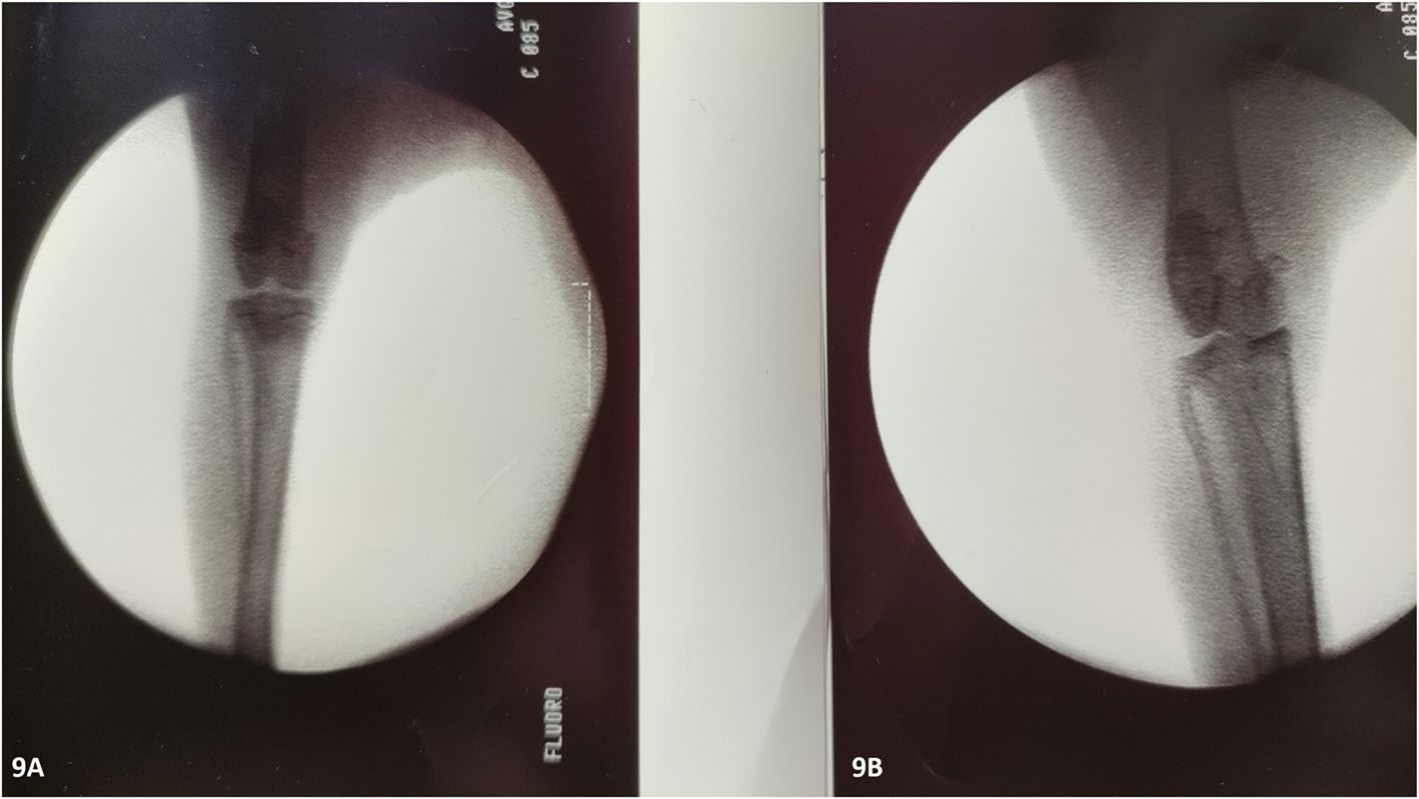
Comparative radiographic images just before (9A) and after 16-weeks post-surgery (9B) showing medial joint space narrowing
